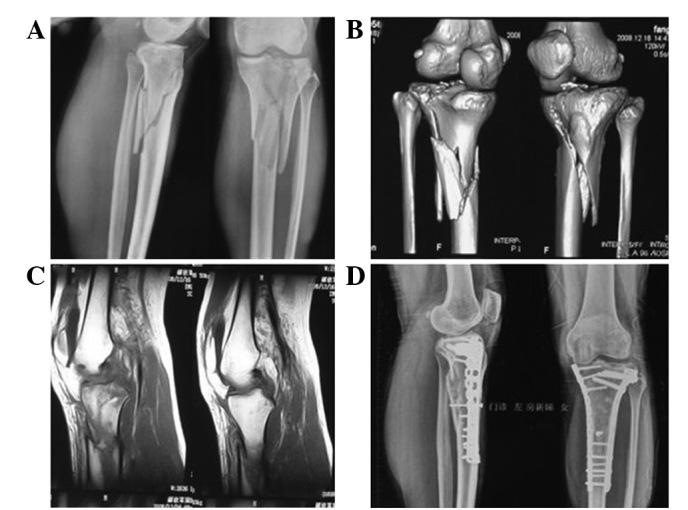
Female, 38 years old, with a serious fracture of the left tibial plateau. (A) Preoperative X-ray. (B) Preoperative computed tomography (CT) and 3D reconstruction showing the serious fracture of the left tibial plateau, with obvious joint surface collapse and fractures of the anterior cruciate ligament. (C) Preoperative magnetic resonance imaging (MRI) showing the fracture of the left tibial plateau. The anterior cruciate ligament was abnormal. (D) One year subsequent to surgery, the X-ray showed that the left tibial plateau fracture fixation was good, and that the joint surface was flat.
